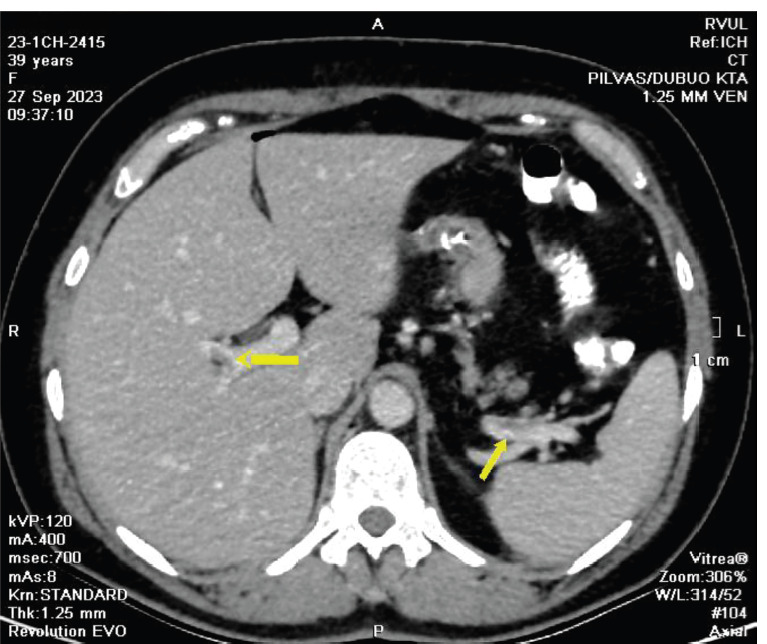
CT scan demonstrates portal and splenic vein thrombosis.
